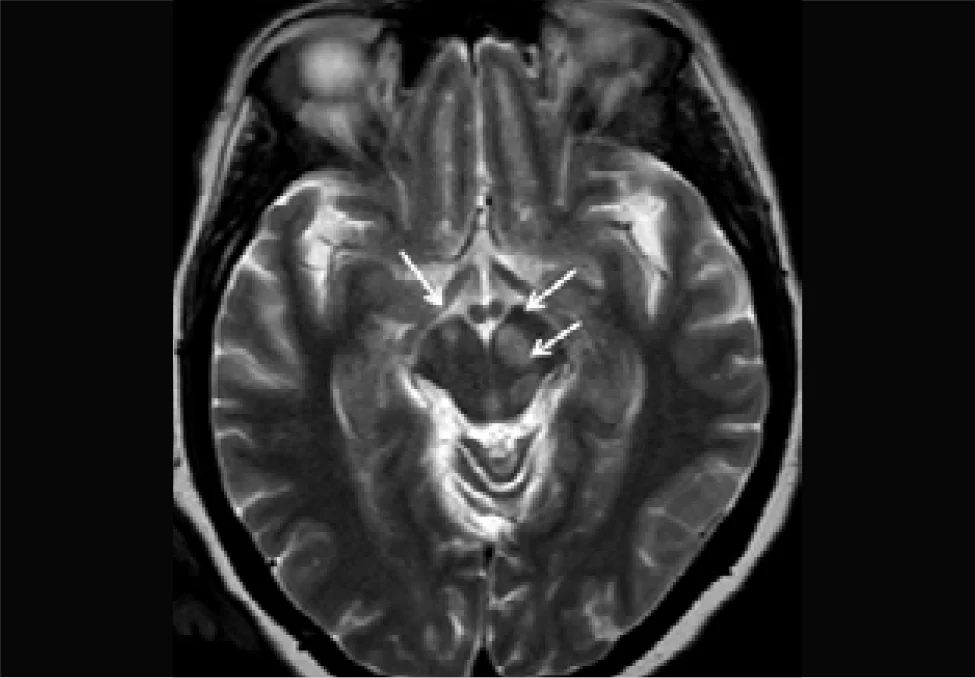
MRI of a 41-year-old man presenting with bilateral pa-resis. Axial T2WI
